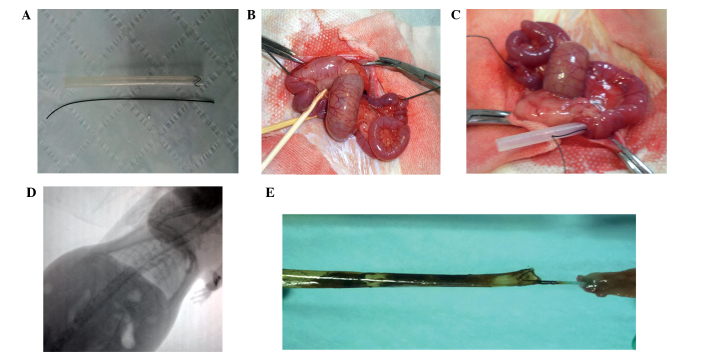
(A) A guide-wire and duodenal-jejunal bypass sleeve (DJBS) used in the study. (B) The guide-wire was introduced from the proximal jejunum and left in the intestinal lumen. (C) The DJBS was released in the duodenum and proximal jejunum. (D) The proximal metal crown was visible in an X-ray. (E) The metal crown of the sleeve remained tightly sutured to the gastric antrum, and the sleeve remained intact without any breakage.
